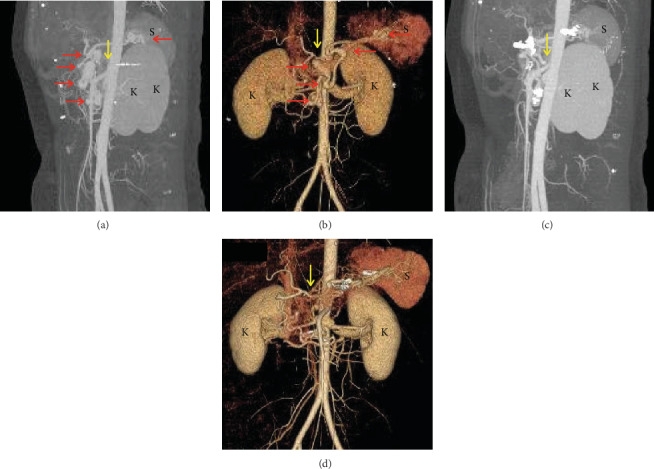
Three-dimensional angiomesenteric computed tomogram of a 37-year-old woman with multiple visceral artery aneurysms (VAAs). (a, b) Preoperative scan showing saccular or fusiform VAAs in the pancreaticoduodenal, dorsal pancreatic, and transverse pancreatic arteries (red arrows). Celiac artery (CA) stenosis with collapsed common hepatic and proximal splenic arteries is visible (yellow arrows). (c, d) On a follow-up scan 66 months after VAA resection and clipping, no recurrence is identified. Note that the collapsed CA branches were reconstituted without the relief of CA stenosis (yellow arrows). K: kidneys; S: spleen.
